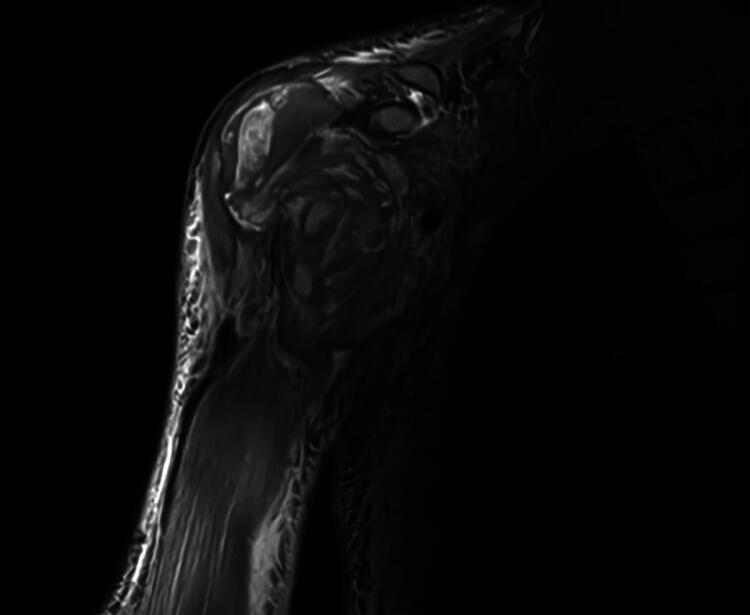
Preoperative MRI showing a heterogeneous, fluid-filled space-occupying lesion in the axilla.
